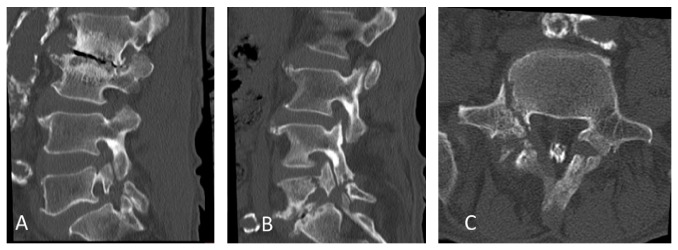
Sagittal reconstruction CT confirming bilateral spondylolysis and destructive changes at L2-L3 and L5-S1 (A, B). Axial CT and right-side parasagittal reconstruction CT confirming right-side L5 pedicle fractures (B, C).
